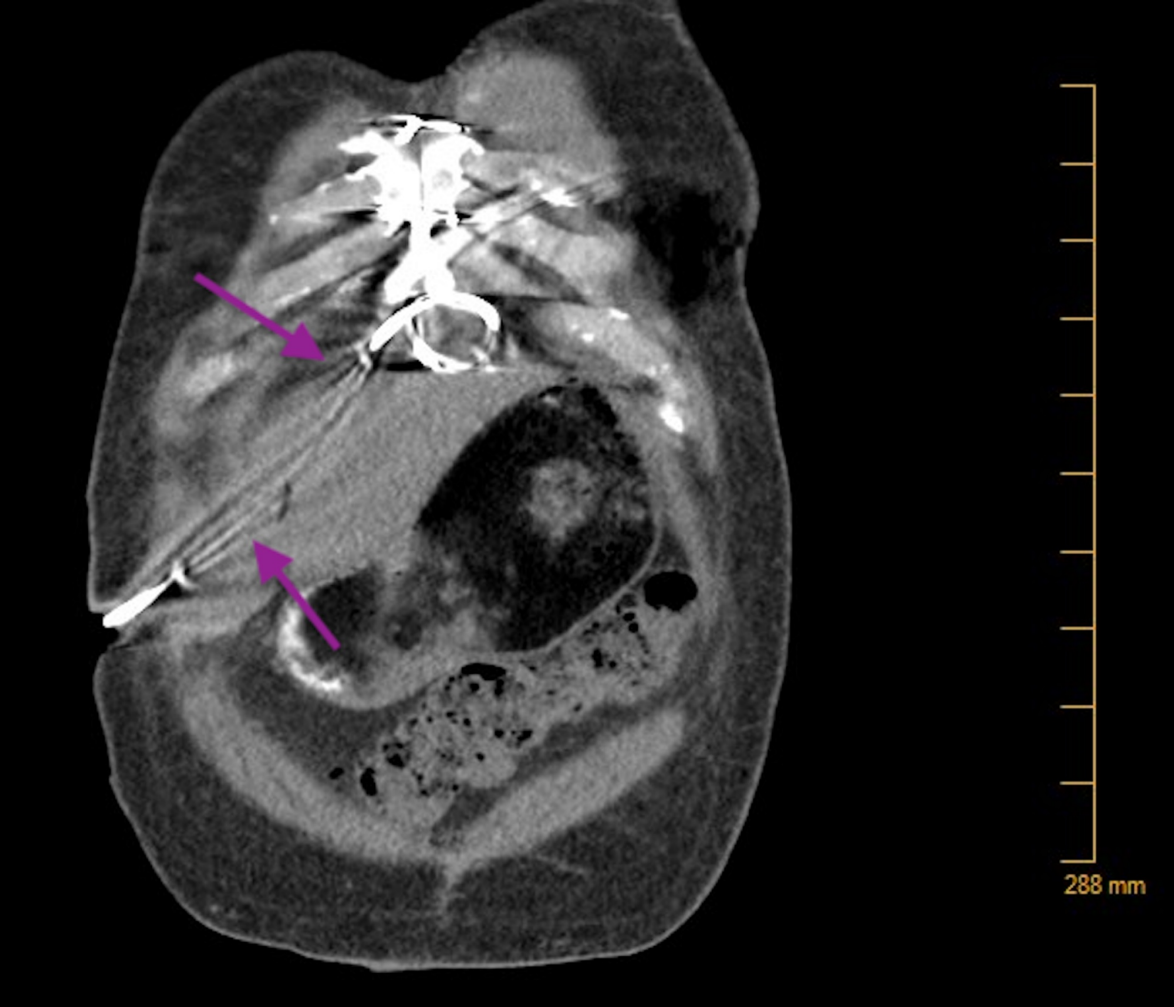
CT Scan of the Abdomen and Pelvis No fluid collection noted along the driveline.
